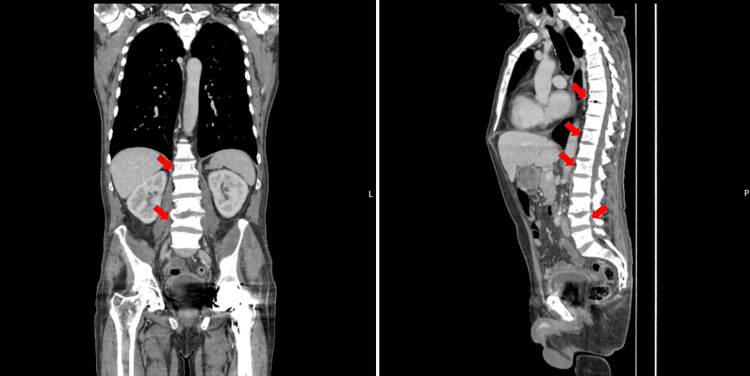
CT TAP showing foci of metastization and flattening of L4 vertebra TAP: Thorax, abdomen, and pelvis
